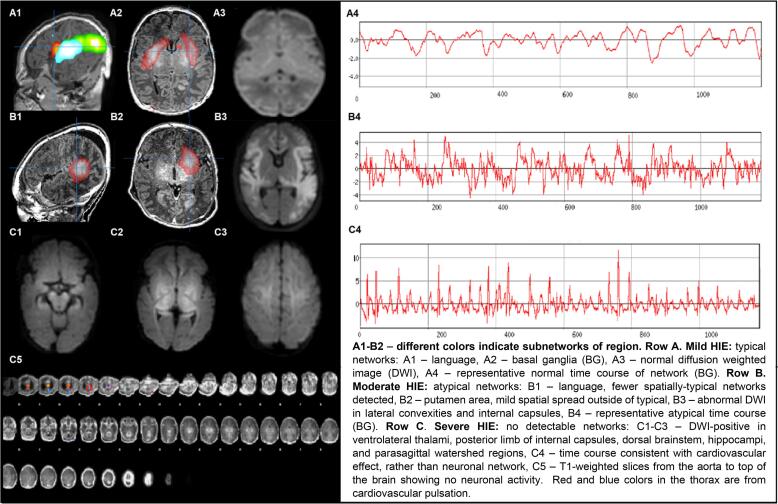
Resting-State Networks (RSN) and Diffusion Weighted Images (DWI) in Neonates with Mild to Severe Hypoxic Ischemic Encephalopathy (HIE).
